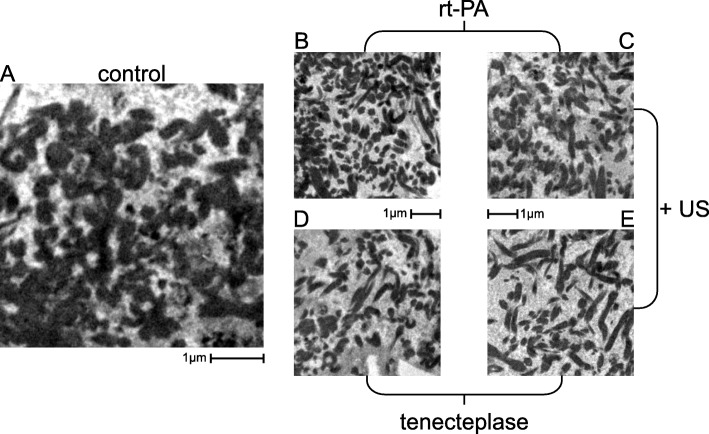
TEM cutouts. Examples of TEM cutouts used for semi quantitative analysis. Treatment group: a control group (solely buffered plasma (pH = 7.4). b rt-PA (50 μg/ml in buffered plasma). c rt-PA (50 μg/ml in buffered plasma) with additional US. d TNK (30 μg/ml in buffered plasma). e TNK (30 μg/ml in buffered plasma) with additional US. US: 2.0 to 2.5 MHz transcranial color-coded ultrasound (spatial-peak temporal-average intensity: 0,179 W/cm^2^; mechanical index = 0.6)
